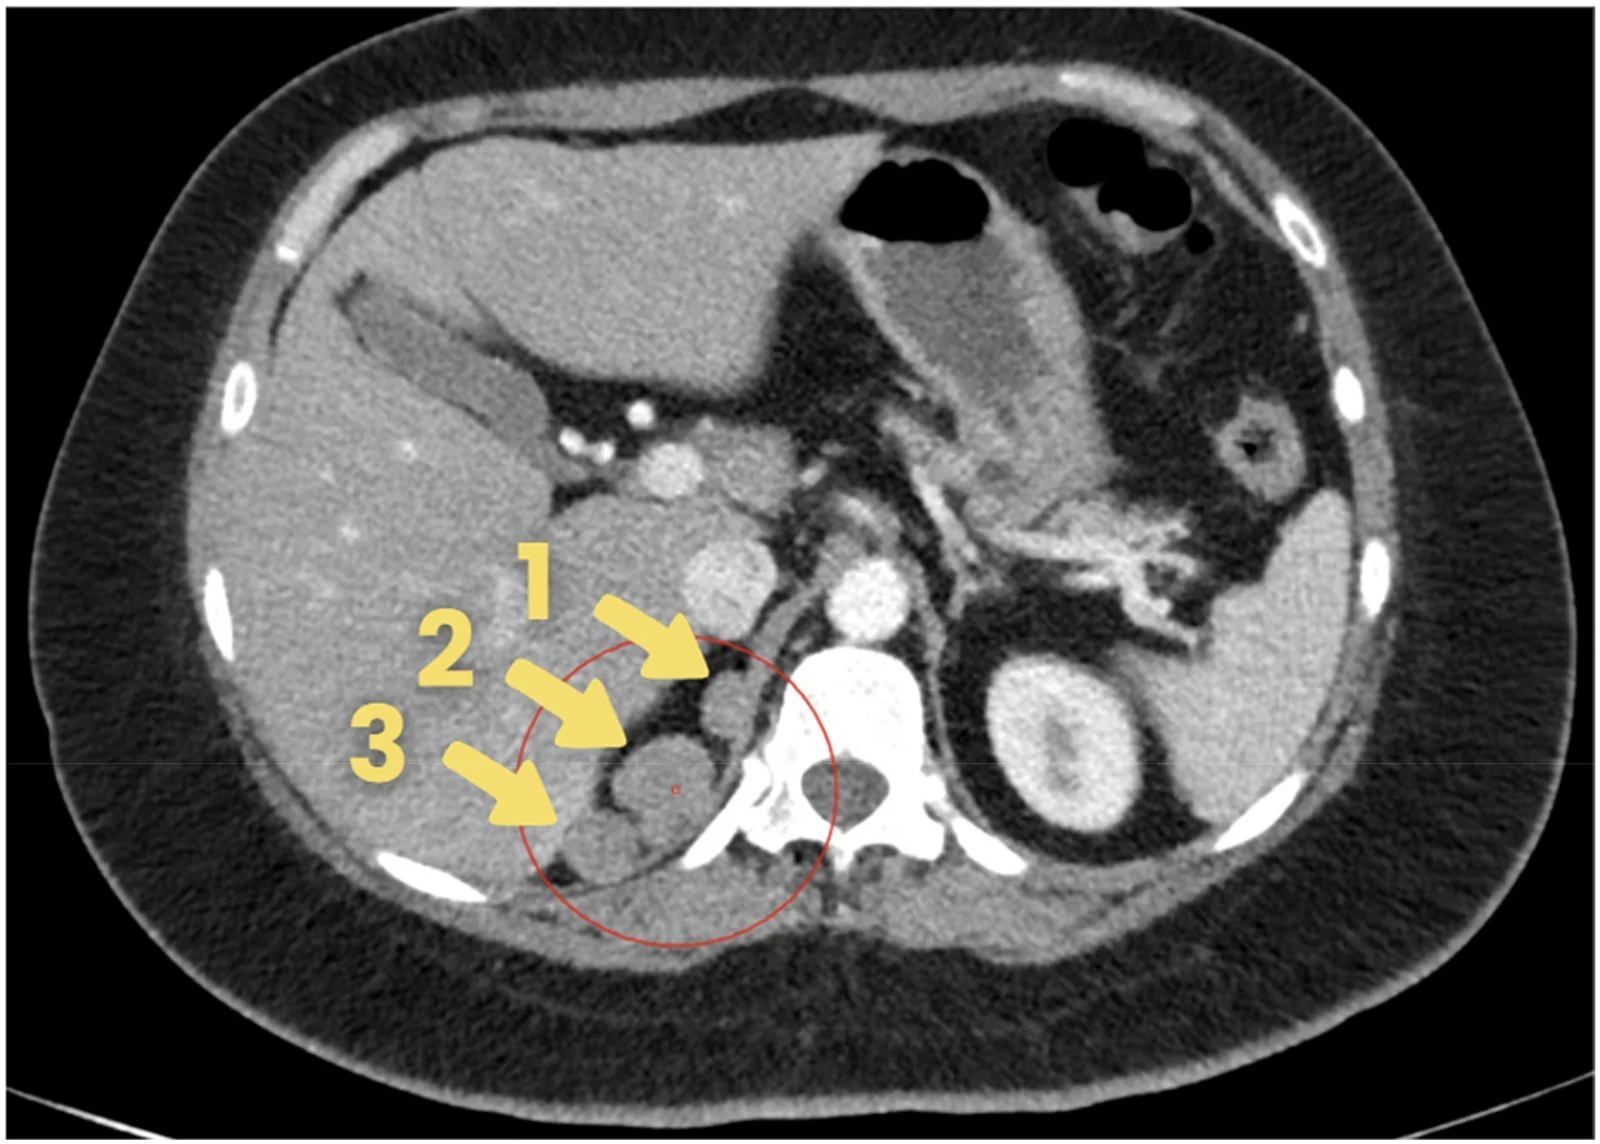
Contrast-enhanced computed tomography (CT) scan of the abdomen showing suspected recurrent right adrenal lesions. Axial image demonstrates at least 3 lobulated, mildly enhancing ovoid lesions in the right suprarenal region (arrows and circled area), measuring 0.9 to 2.0 cm, suspicious for recurrence. Quantitative assessment shows: (1) 49 Hounsfield unit (HU) with 26% washout, (2) 39 HU with 37% washout, and (3) 36 HU with 23% washout.
